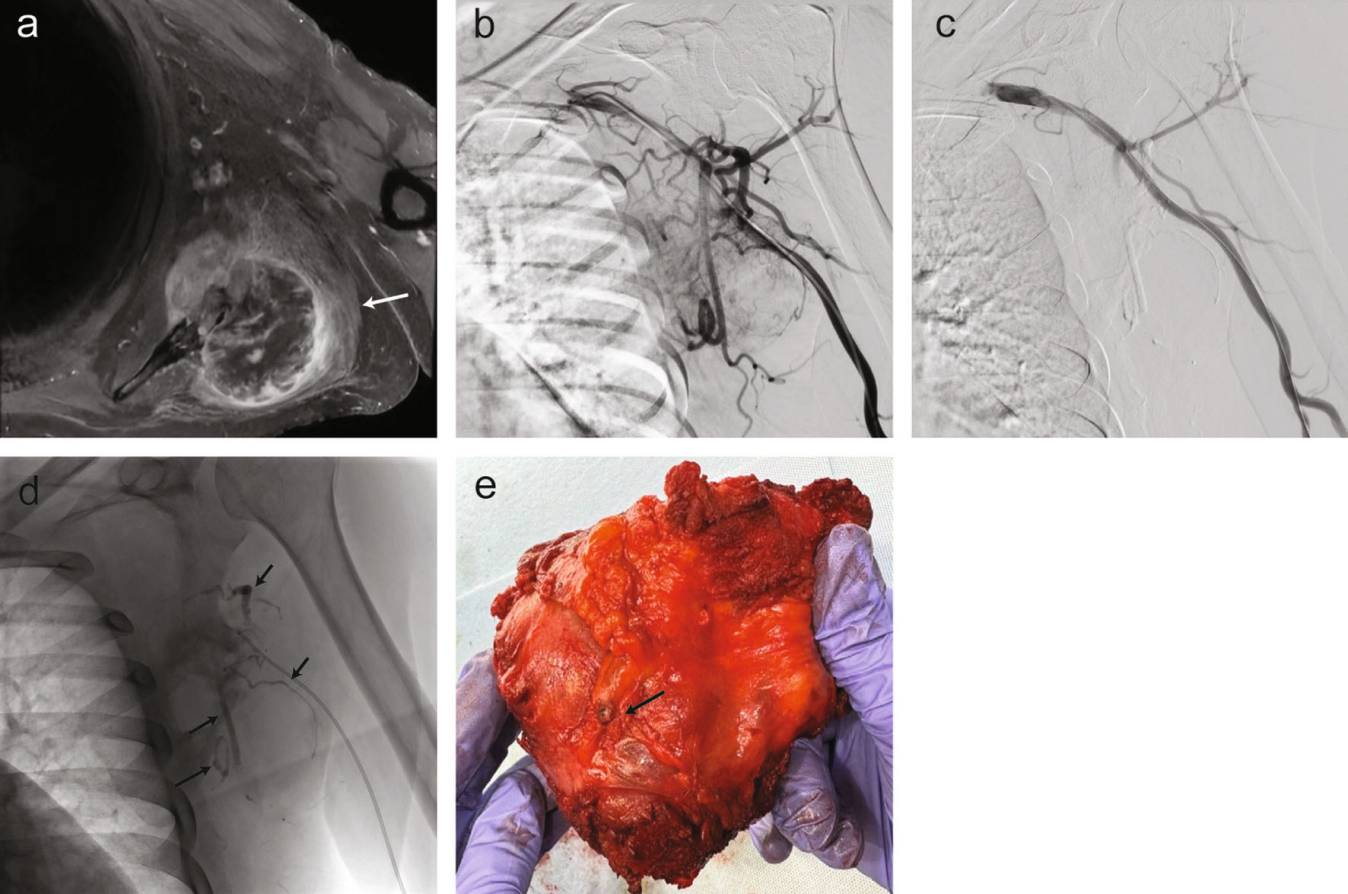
Preoperative embolization of a scapular mass in a 66-year-old male with a history of pT3aN1M1 clear cell renal cell carcinoma. (A) Axial T1-weighted image with gadolinium administration shows an enhancing mass centered at the left scapula (arrow). (B) Pre-embolization left axillary artery angiography demonstrating tumor blush originating from multiple branches of the long thoracic artery. (C) Post-embolization angiography via a 4 French angled catheter with a total of 1.4 cc CE delivered via 2.8 a French microcatheter demonstrating devascularization of the lesion. (D) Post-embolization fluoroscopic image demonstrating conformable embolic casts within the area of prior tumor blush (arrows). (E) Gross pathology following same-day radical resection of the metastatic renal cell carcinoma mass demonstrating occlusion of vascular supply with CE (arrow). The lesion was found to be a multilobulated tan-pink necrotic mass measuring 6.2 × 4.2 × 3.9 cm
